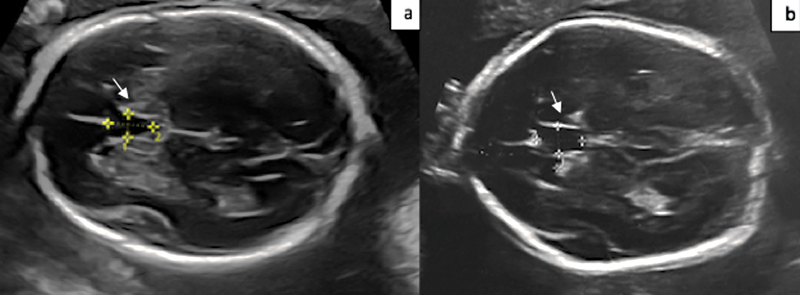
Two-dimensional ultrasound images of the cavum septi pellucidi (white arrow) in a euploid fetus (a) and in a fetus with trisomy 18 (b).
